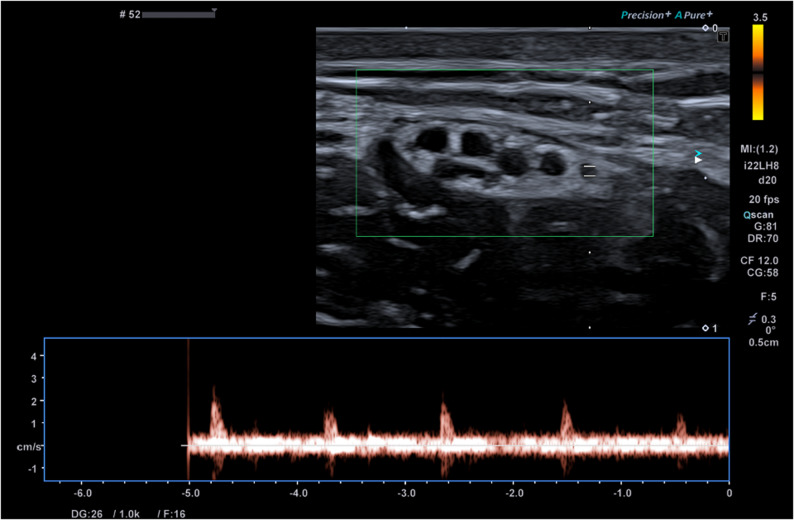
Axial section of pulsed wave Doppler ultrasound as a noninvasive method for estimating pulse wave velocity of the ulnar arterial vascularization
